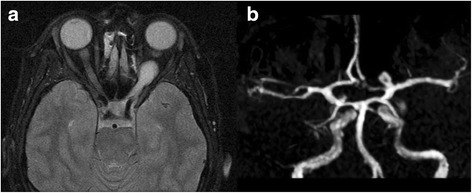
Preoperative magnetic resonance imaging showing, in T1-weighted sequence, the left optic glioma (a) and, in time-of-flight sequence, a suspect aneurysmatic dilatation (6×5 mm) on the left carotid bifurcation (b)
